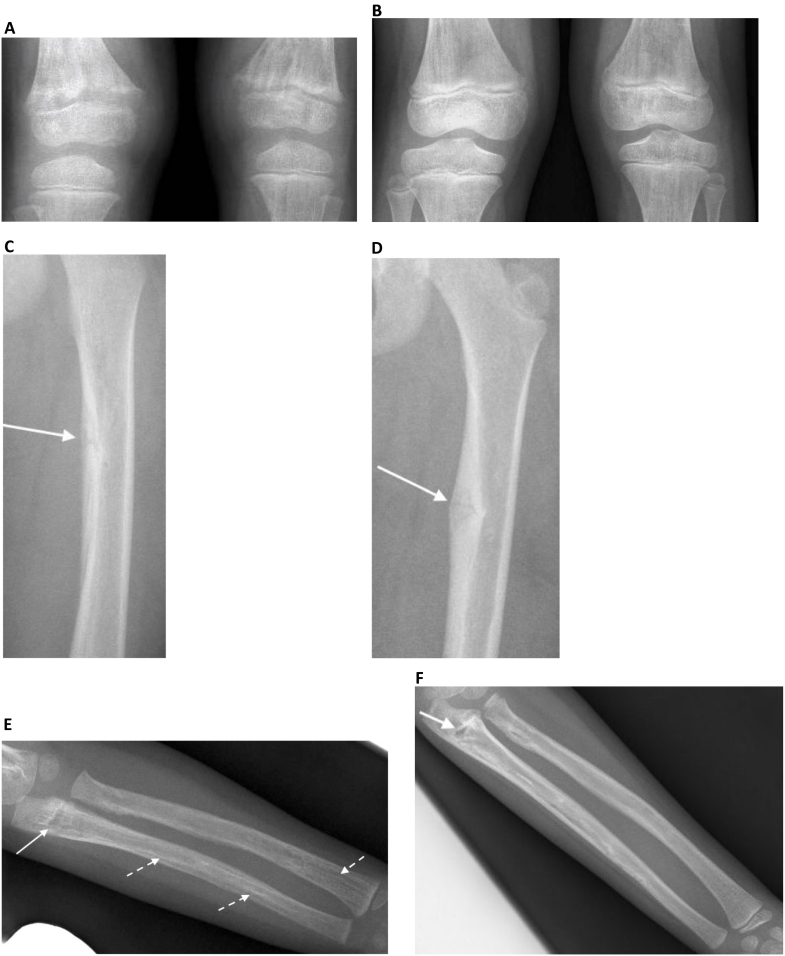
Radiographic comparison of patient's condition after 10 months of conventional therapy and before treatment with burosumab. A. Radiographs of the knees before treatment with burosumab. Widening and fraying of the distal femoral metaphyses are consistent with rickets. Moreover, the sub-metaphyseal areas of lucency and vertical cortical striations are clearly visible in the left distal femoral radiograph. B. Radiographs of knees 12-months after treatment with burosumab. Radiograph of knees showing healing of radiological signs of rickets and improvement in bone texture. C. Radiograph of the left proximal femur before treatment with burosumab. Note the left medial cortical pseudofracture (arrow), with periosteal reaction. D. Radiograph of the left proximal femur 12 months after treatment with burosumab. Note cortical thickening and partial healing of left medial cortical pseudofracture (arrow). E. Radiograph of the left forearm before treatment with burosumab. Note the proximal ulnar pseudofracture (arrow). Also shown are diaphyseal areas lucency and sclerosis (dashed arrows). F. Radiograph of the left forearm 12-months after treatment with burosumab. Note the partial healing of the proximal ulnar pseudofracture (arrow) and improvement in the diaphyseal bone texture.
